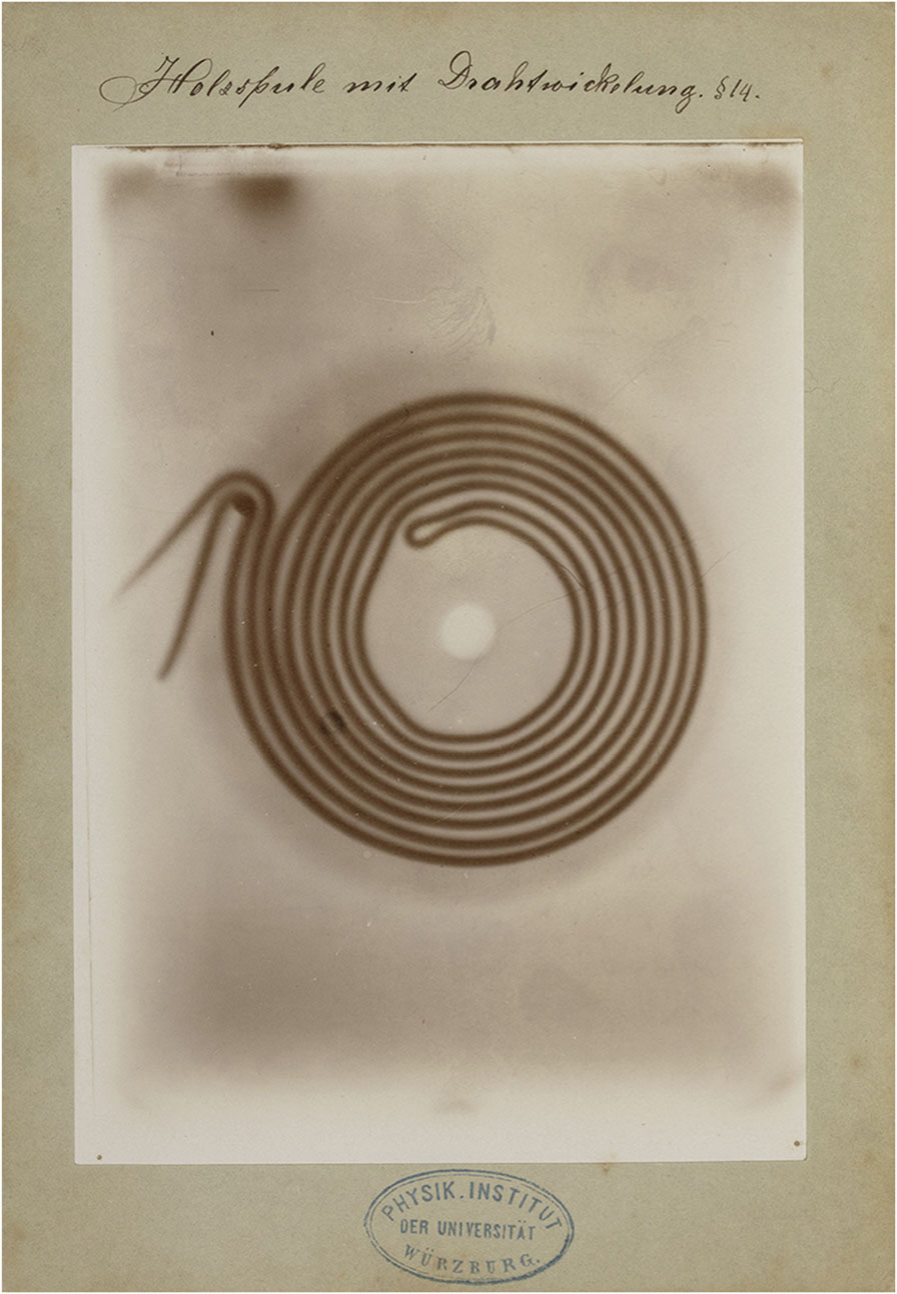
A wooden take-up spool with wire. This image shows that the wood of the spool is practically transparent to the X-rays while the wire is not. Manually written legend: ‘Holzspule mit Drahtwickelung’ (Wooden take-up reel with wire). Paragraph referral: § 14
